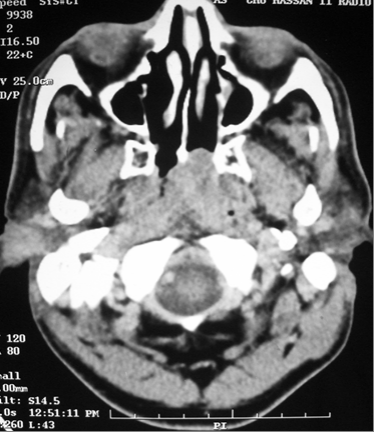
Computed tomography scan before treatment. Left nasopharyngeal mass involving the parapharyngeal space but without extracranial or bone involvement.
